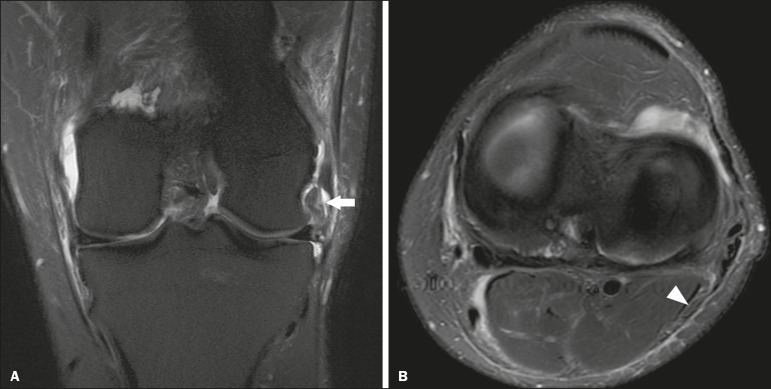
A 43-year-old male patient who suffered trauma playing soccer. A: Coronal T2-weighted MRI sequence with fat saturation, showing PLC lesion, highlighting the tear in the popliteus tendon (arrow). There was also injury to the joint capsule and the LCL (not shown). B: Axial T2-weighted MRI sequence with fat saturation, showing the CPN (arrow), which was considered normal in this case.
